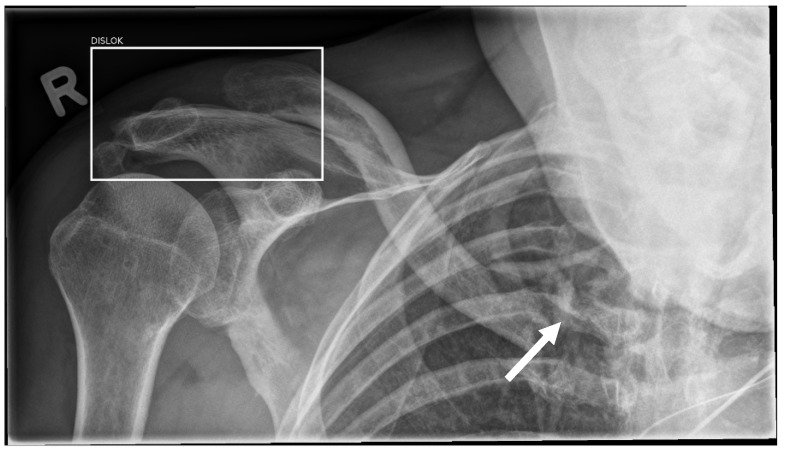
Standing ap radiograph of the right shoulder. The AI software correctly sets a bounding box around the acromioclavicular joint injury (marked as “Dislocation”); however, both the AI and the resident missed the displaced proximal clavicle fracture (white arrow).
